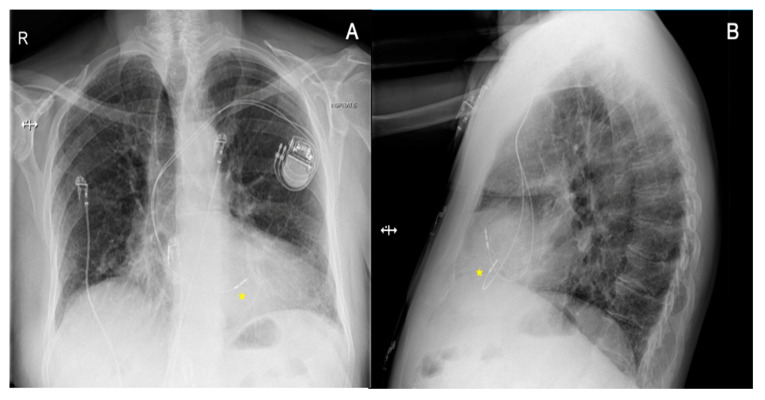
Chest X-rays of a patient after transcatheter aortic valve implantation who developed a complete atrioventricular block and received a pacemaker with conduction system pacing. A posterior-anterior view (panel A) and a lateral view (panel B). An atrial lead was placed in the right atrial appendage, while a ventricular lead was placed in the left bundle branch area pacing site (indicated by asterisk).
